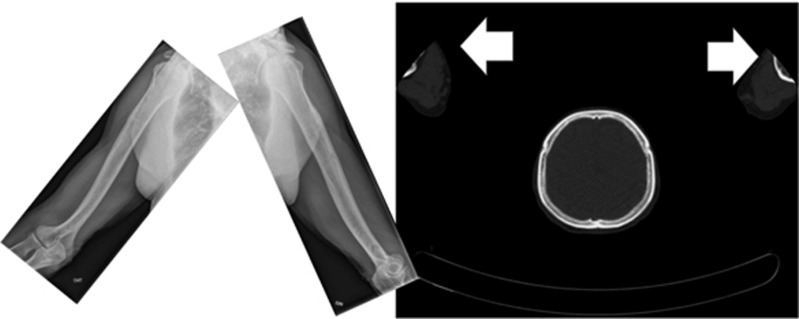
X-rays (left) and CT (right) showing the limited field of view for some CT protocols in which the arms had been placed above the head. In such cases, the elbows protruded outwards, and as a result, the distal humeri were either ‘cut off’ or lay in the field periphery where the image quality of many CT scanners is limited.
